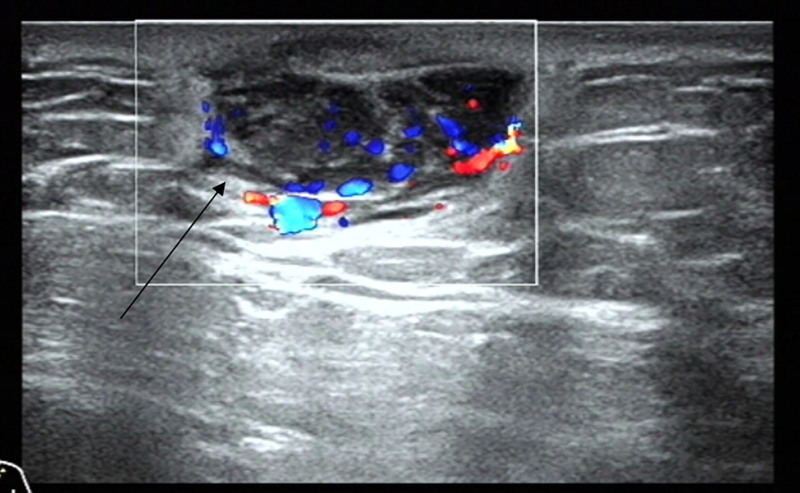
Ultrasound of the left breast showed a mixed echogenic mass in the subcutaneous fat layer of the left breast with rich blood supply.
